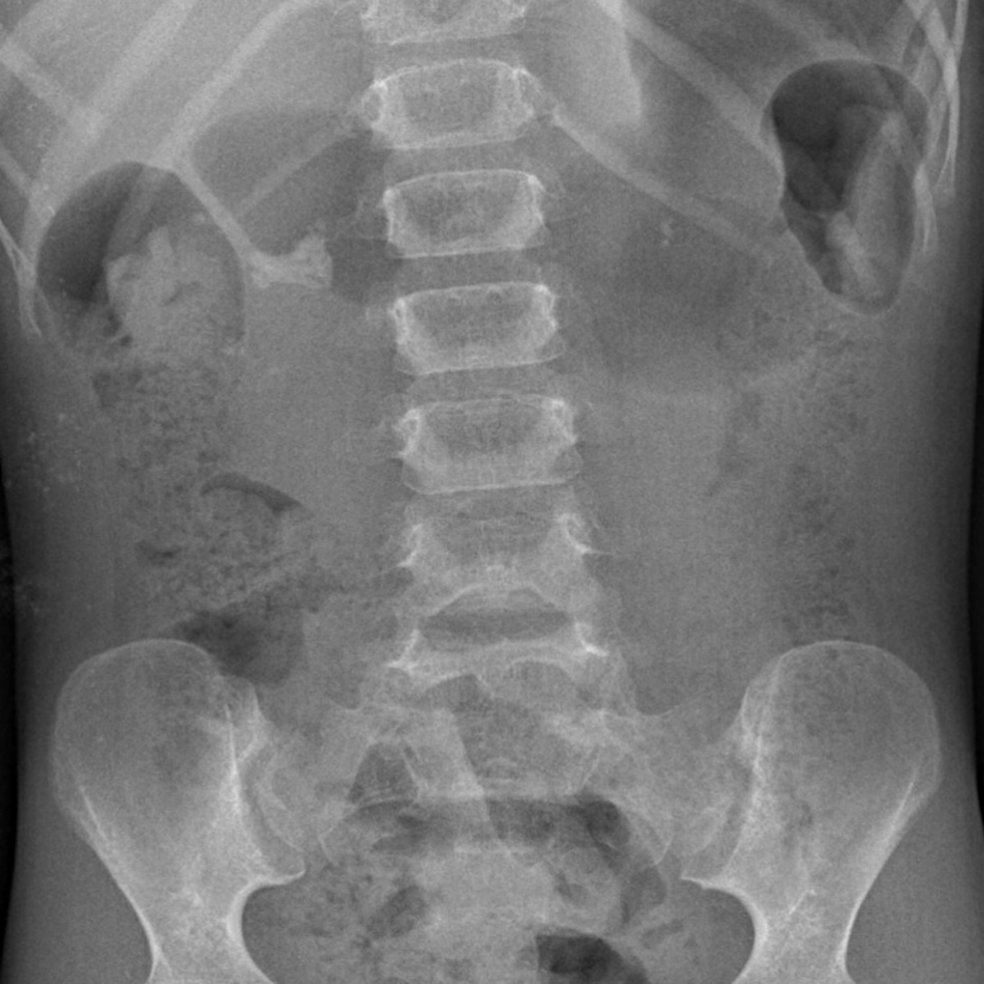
Preoperative plain X-ray image showing a partial staghorn calculus.
